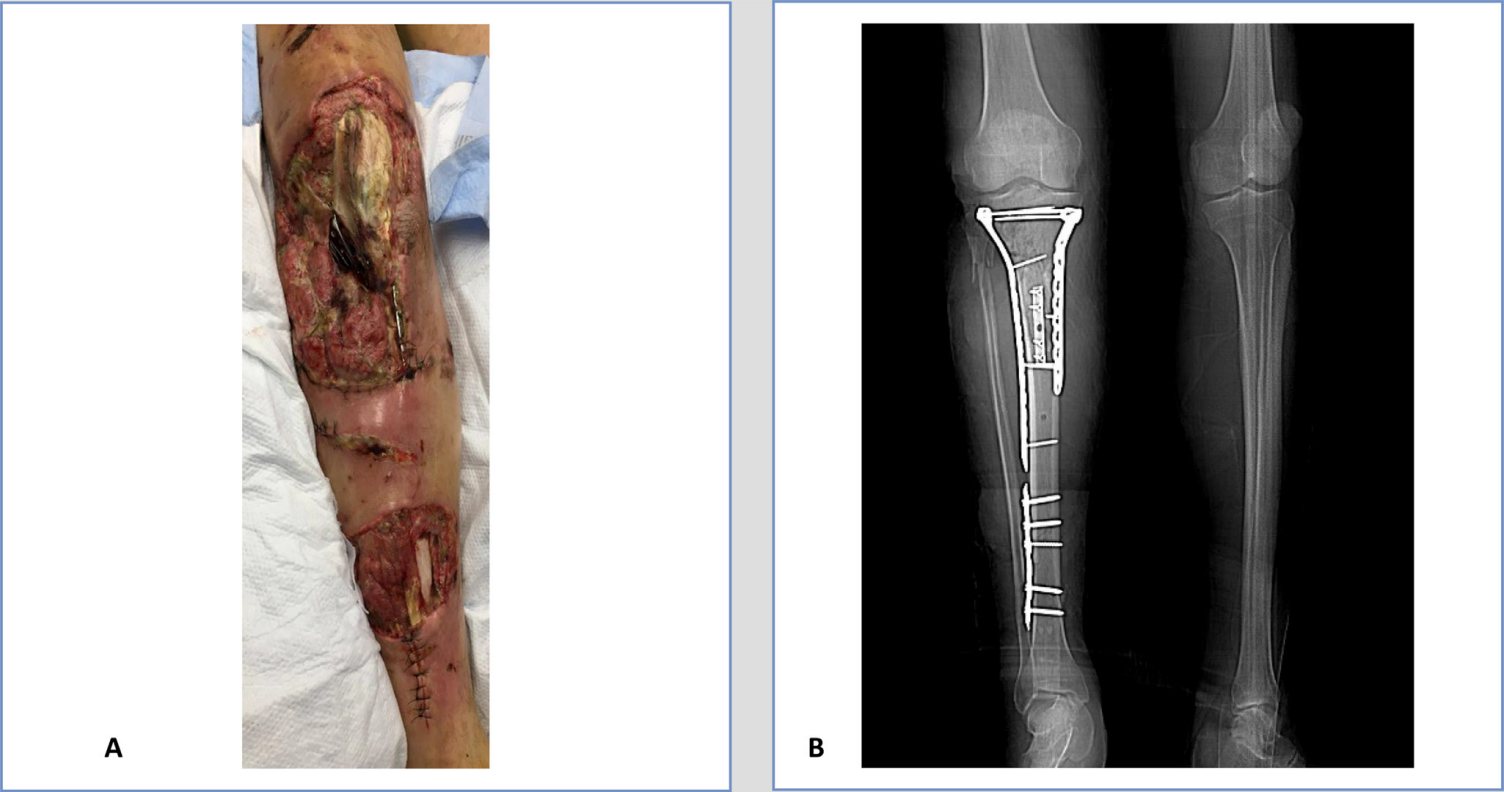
(A). The clinical photograph and (B) the radiograph of the right open tibial-fibular fracture status post open reduction internal fixation (ORIF) with overlying wound and exposed hardware at day 7 after the firework explosion accident.
